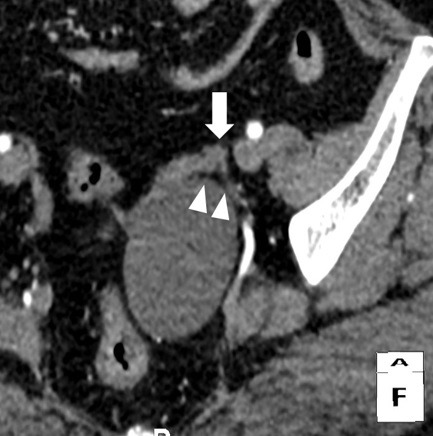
A CT multiplanar reconstruction (MPR) image shows linear fat planes (white arrowhead) between the left ovary (white arrow) and the cyst
